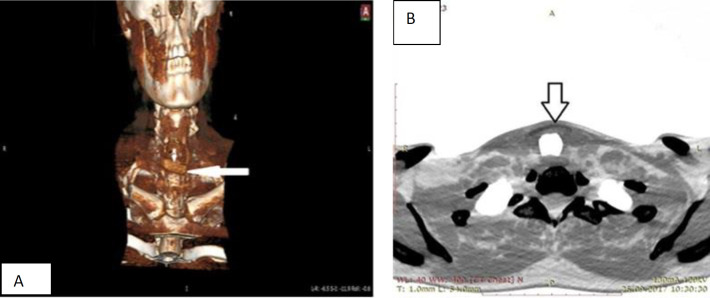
CT scan of neck organs in 2 frontal (a) and axial (b) projections 6 months after plastic closure LTD: cartilaginous autograft is indicated by an arrow
